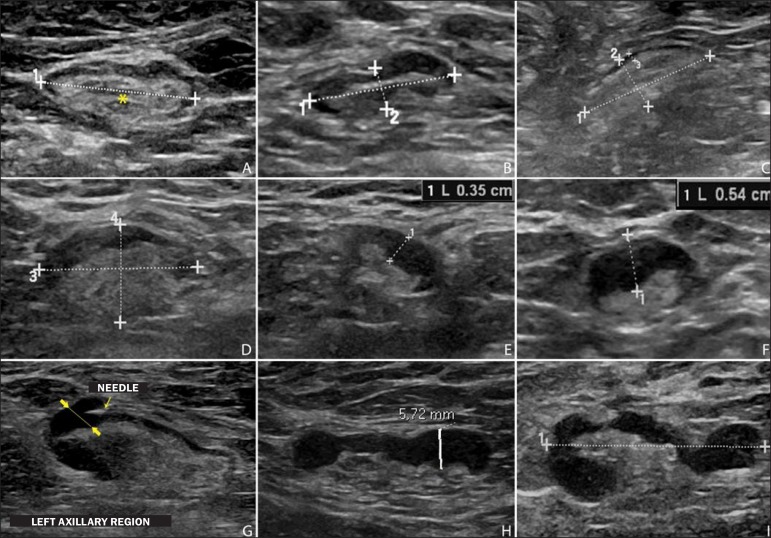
Sonographic images demonstrating some lymph nodes classified as
